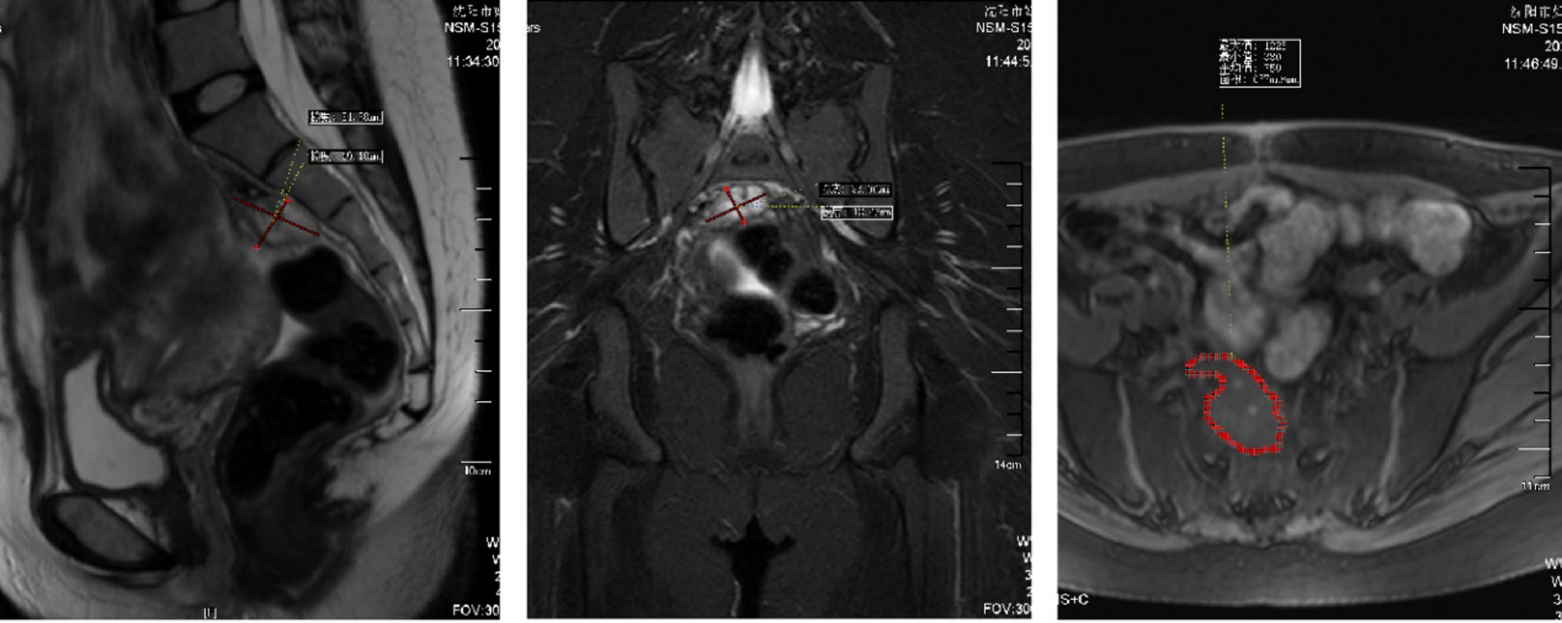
Follow-up MRI at 60 days postpartum. A mixed-signal mass (33 mm×29 mm×19 mm) located in the retroperitoneal space posterior to the lower uterus, consistent with an organized hematoma
